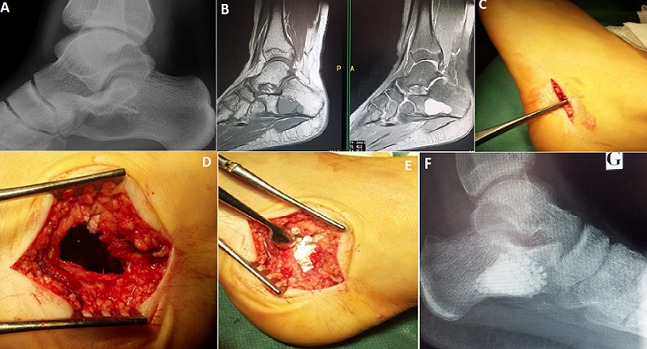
(A) radiographie standard du pied gauche montrant une image lacunaire ostéolytique du calcanéum comportant de fines cloisons avec corticale amincie; (B) IRM du pied gauche: formation liquidienne polylobée bien limitée interessant les tiers antérieur et moyen du calcanéum en hyposignal modéré en T1 et hypersignal liquidien en T2 associé à un niveau liquidien postérieur faisant suspecter une composante hémorragique; (C) image péropératoire: répérage scopique de la voie d’abord; (D) image péropératoire: trépanation de la face externe de l’os et curetage évidement de la cavité; (E) image péropératoire: comblement de la cavité par une greffe à partir de subtituts osseux; (F) contrôle radiologique post opératoire à un mois
